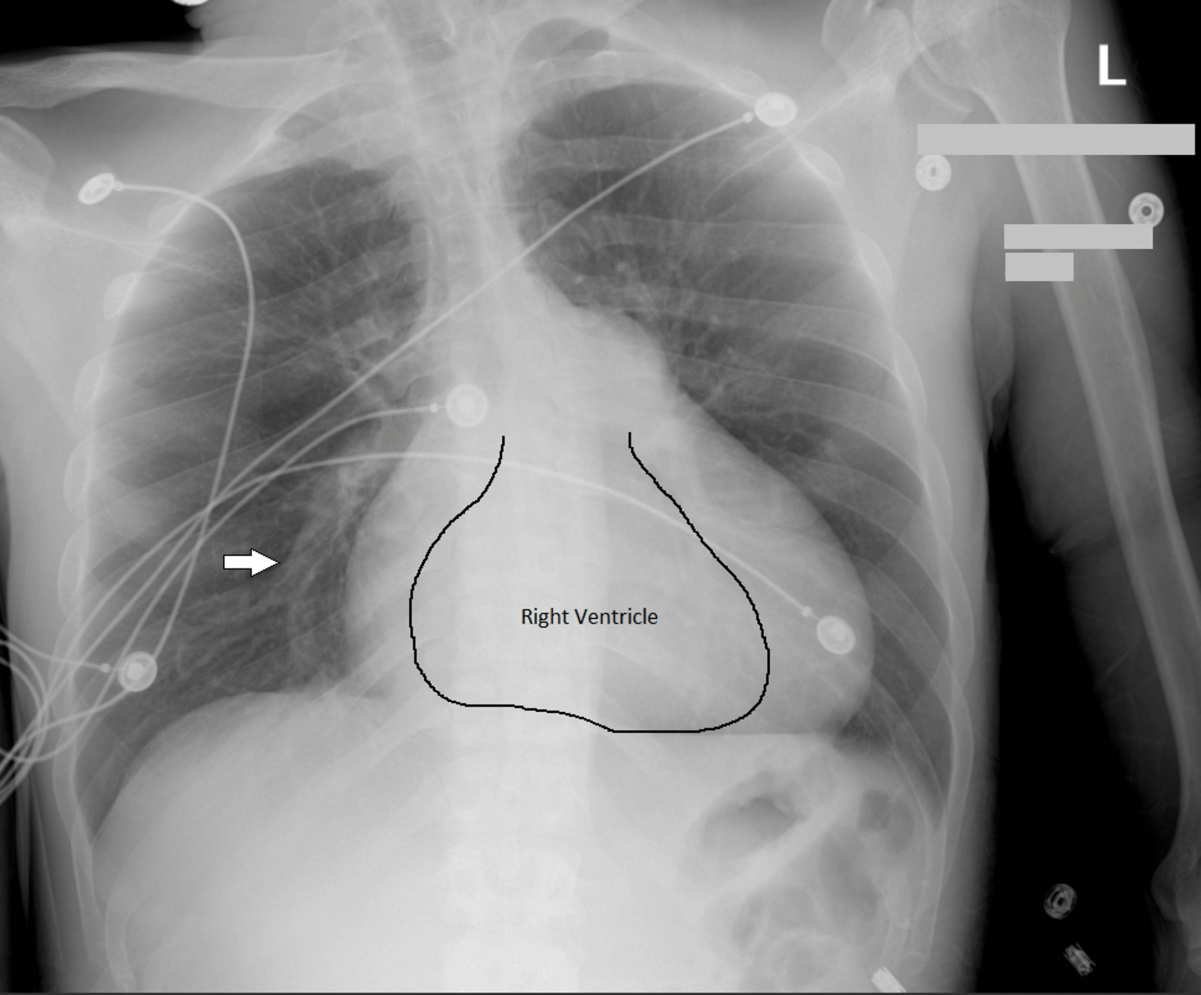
The chest X-ray shows abnormal enlargement of the main pulmonary artery. The white arrow points at the main pulmonary artery.
